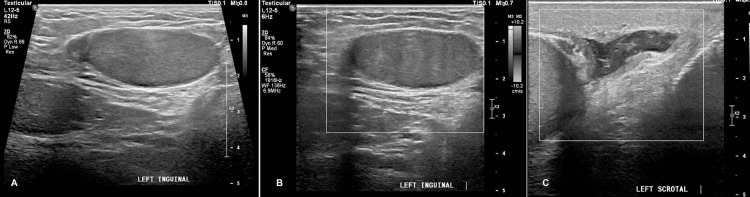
Preoperative Doppler ultrasound - case 2 The images show the left testis in the left inguinal canal (panels A, B) with no vascularity. Panel C depicts the scrotal sac
